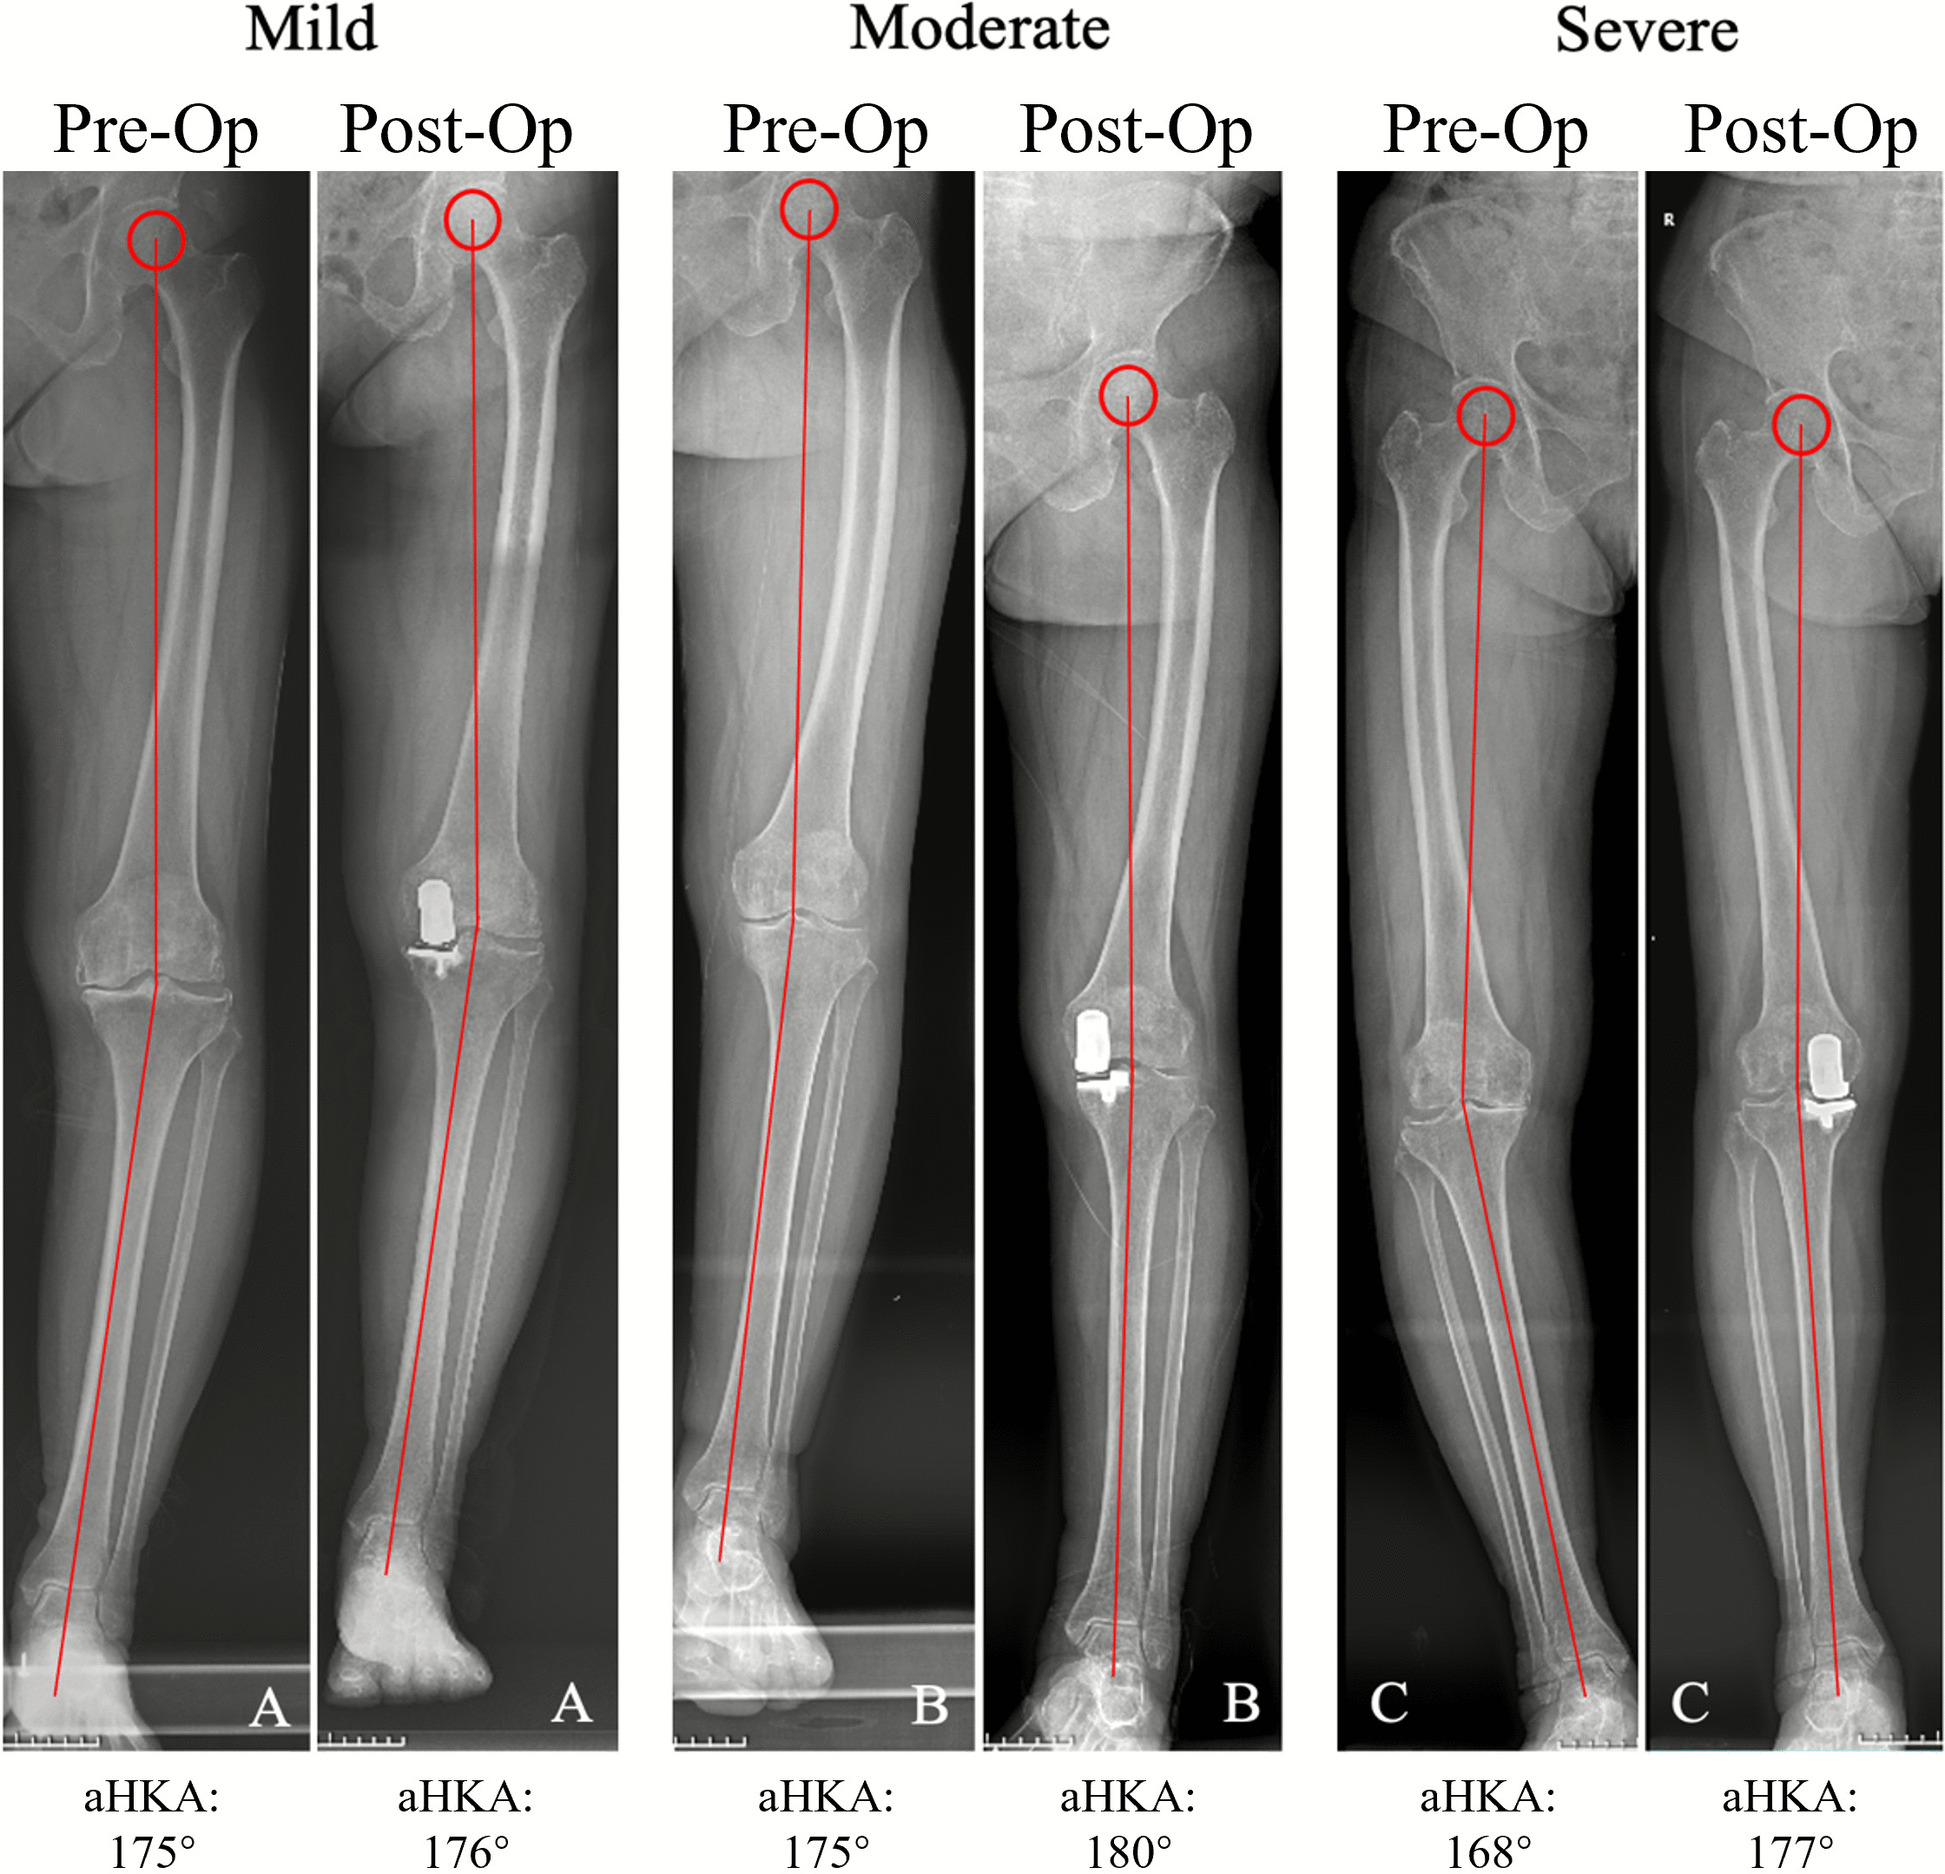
Examples of radiographic assessment of aHKA from different peri-operative varus alignment changes: A: mild varus alignment change (≤ 4°), B: moderate varus alignment change (> 4° and < 7°), C: large varus alignment change (≥ 7°)
